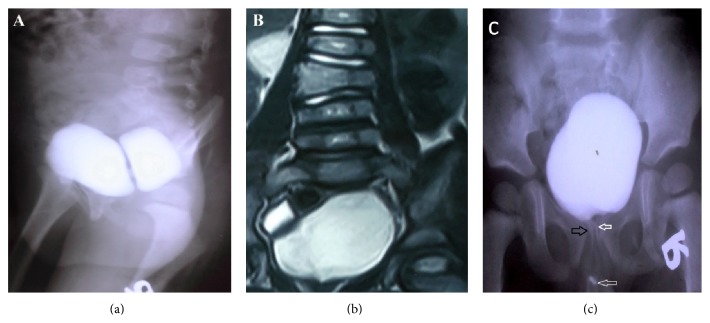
Voiding cystourethrogram reveals bladder duplication in coronal plane (sagittal view) (a). Spinal magnetic resonance imaging demonstrates butterfly vertebrae in L1, L2, and L3 (b). Voiding cystourethrogram shows two urethral meatus originating from main bladder with the dorsal stenotic (black arrow) and patent ventral urethra (white arrow) (coronal view) (c).
